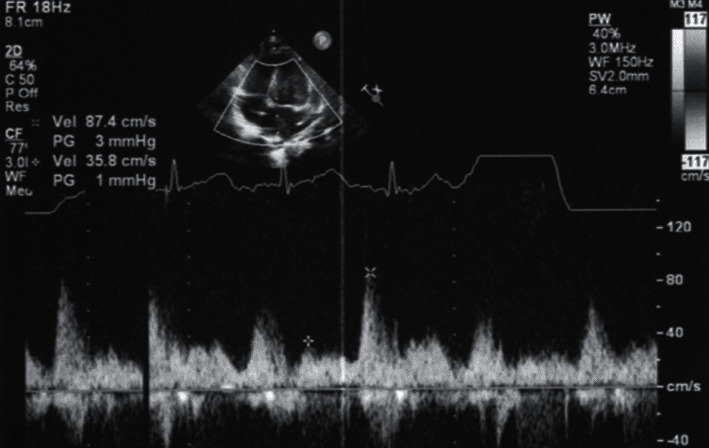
Echocardiography at the age of 4 months in the outpatient clinic. Inflow of blood from the common chamber to the left atrium can be observed. The flow pattern is pulsatile, and no anastomotic stenosis is observed. The cardiac contractility is good, and no other abnormal findings, including pulmonary vein stenosis, are observed.
